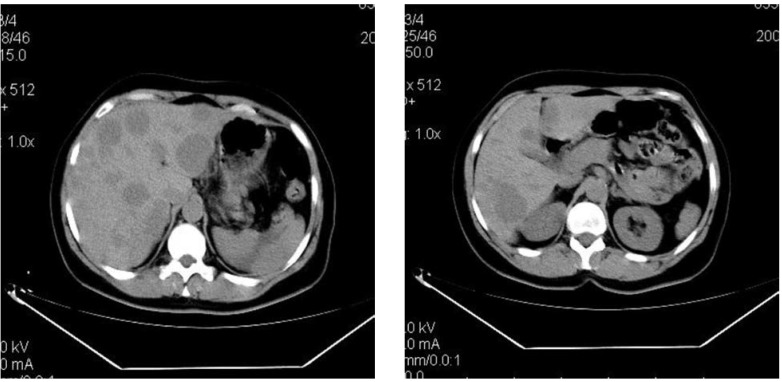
Abdominal CT ( june 2006) revealed a 1.5 cm mass localized at the head of pancreas and diseminated hepatic tumors
